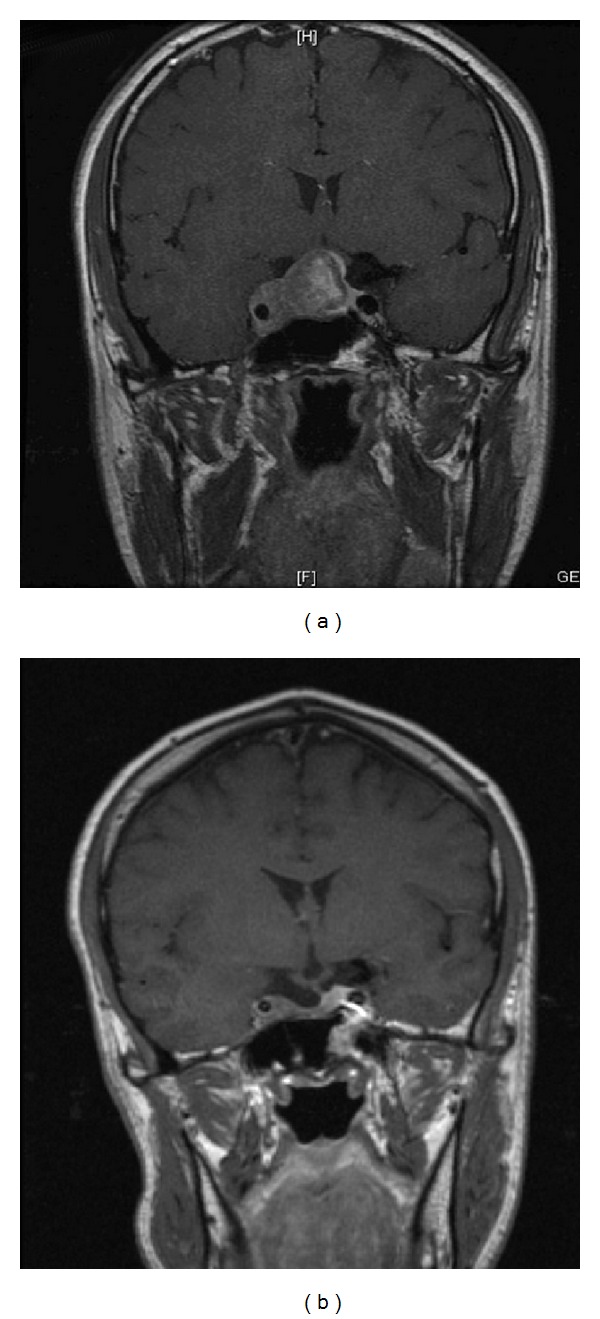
Pretreatment and postcabergoline MRI showing resolution of right-sided cavernous sinus invasion.
